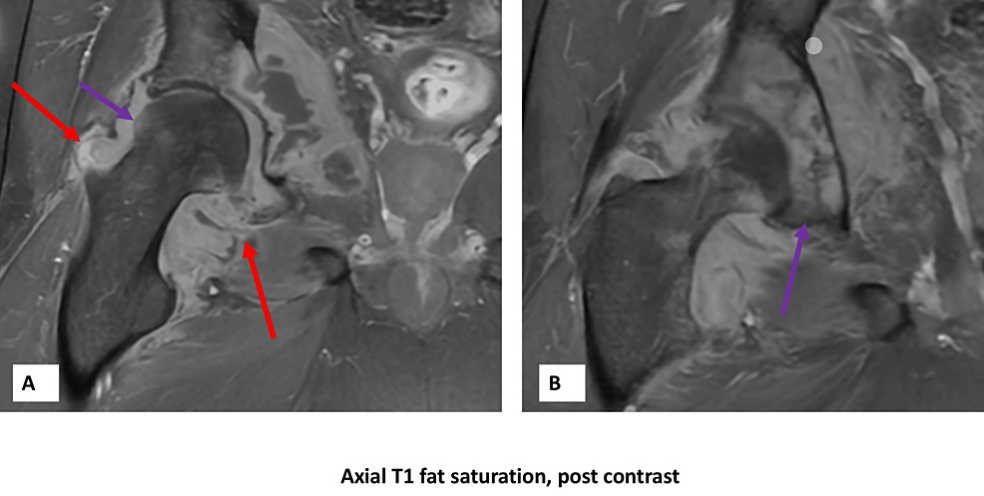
MRI of the right hip after contrast administration. (A) Enhancement of the joint fluid (red arrows), as well as the acetabulum (purple arrows), suggestive of septic arthritis and osteomyelitis, respectively. (B) Enhancement of around the acetabulum (purple arrow).
